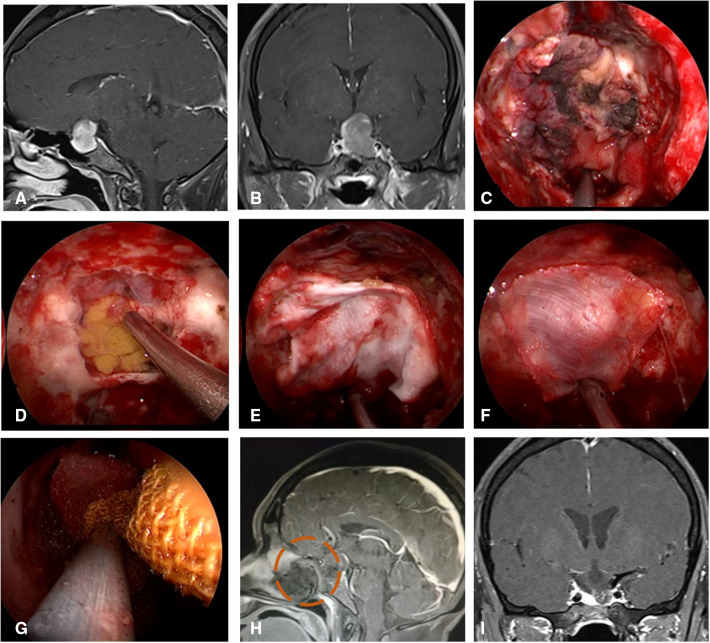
Repair process of patient with VP-NSF inactivation. (A,B) MRI enhanced in sellar region before operation and was considered pituitary adenoma. (C) VP-NSF inactivation observed on the endoscopy (black area). (D) Unraveling the artificial inner dura. (E) Artificial dura mater embedded between cellulite and dura mater. (F) Replacement of inactivated nasal septal mucosal flap using fascia lata. (G) Collagen sponge and biological protein glue was fixed and then supported with iodoform gauze. (H) MRI enhancement at postoperative week 2 showed no significant enhancement of VP-NSF (circle). (I) Postoperative MRI enhancement in sellar region. VP-NSF, vascularized pedicle nasoseptal flap.
